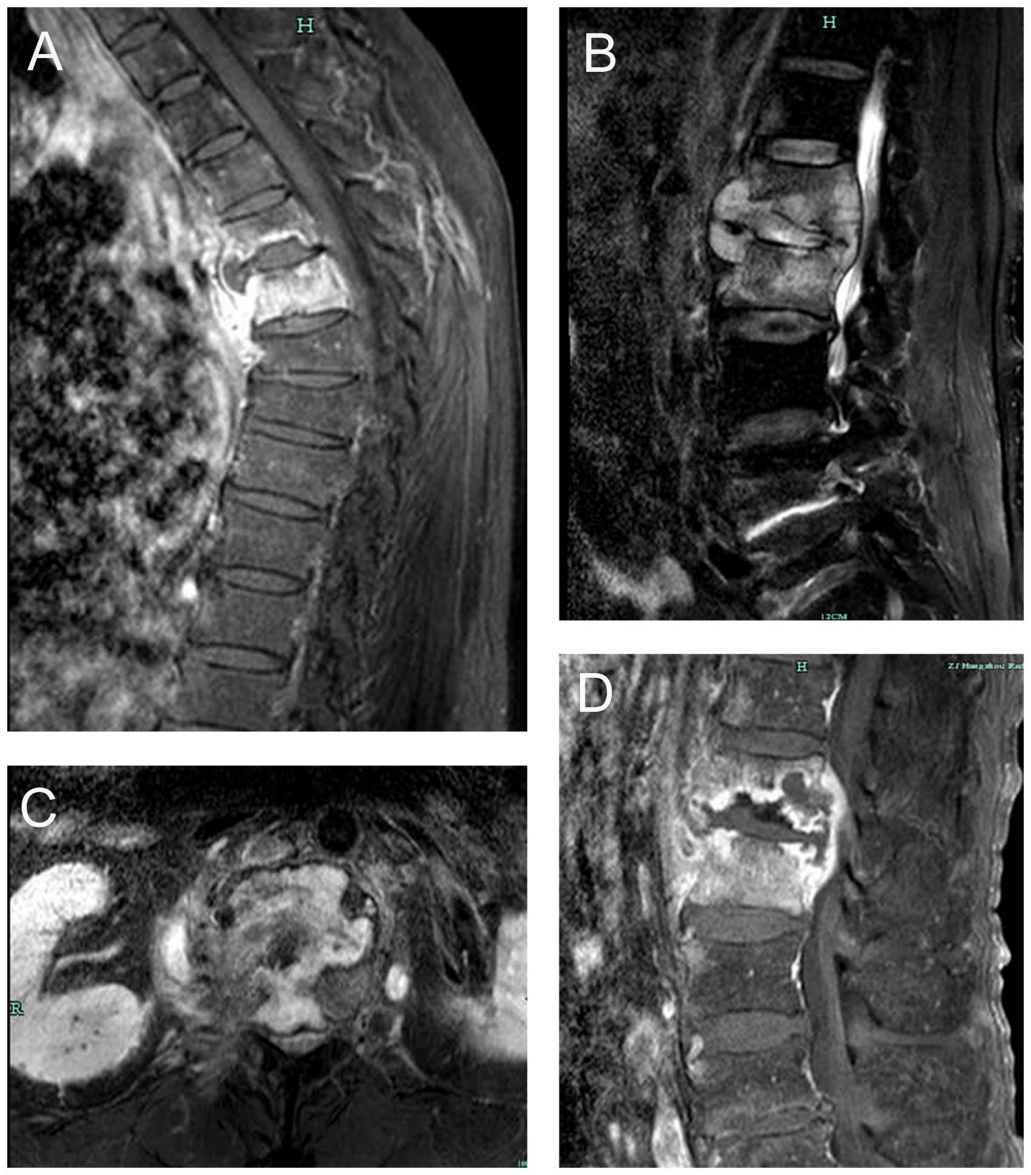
Magnetic resonance imaging changes of spinal tuberculosis. (A) thoracic tuberculosis leading to destruction of vertebral body and prevertebral abscess. (B) lumbar tuberculosis leading to destruction of vertebral body, intervertebral disk and prevertebral abscess. (C) lumbar tuberculosis leading to destruction of lumbar vertebral body. (D) lumbar tuberculosis leading to worm-bite like destruction of vertebral body and intervertebral disk.
